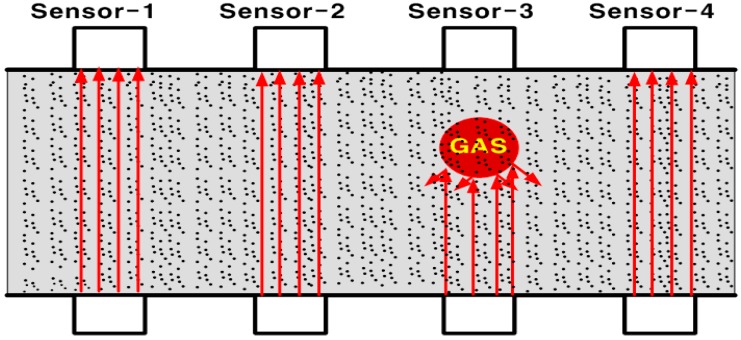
Example of sludge measurement distortion using an ultrasonic multi-beam sensor.
